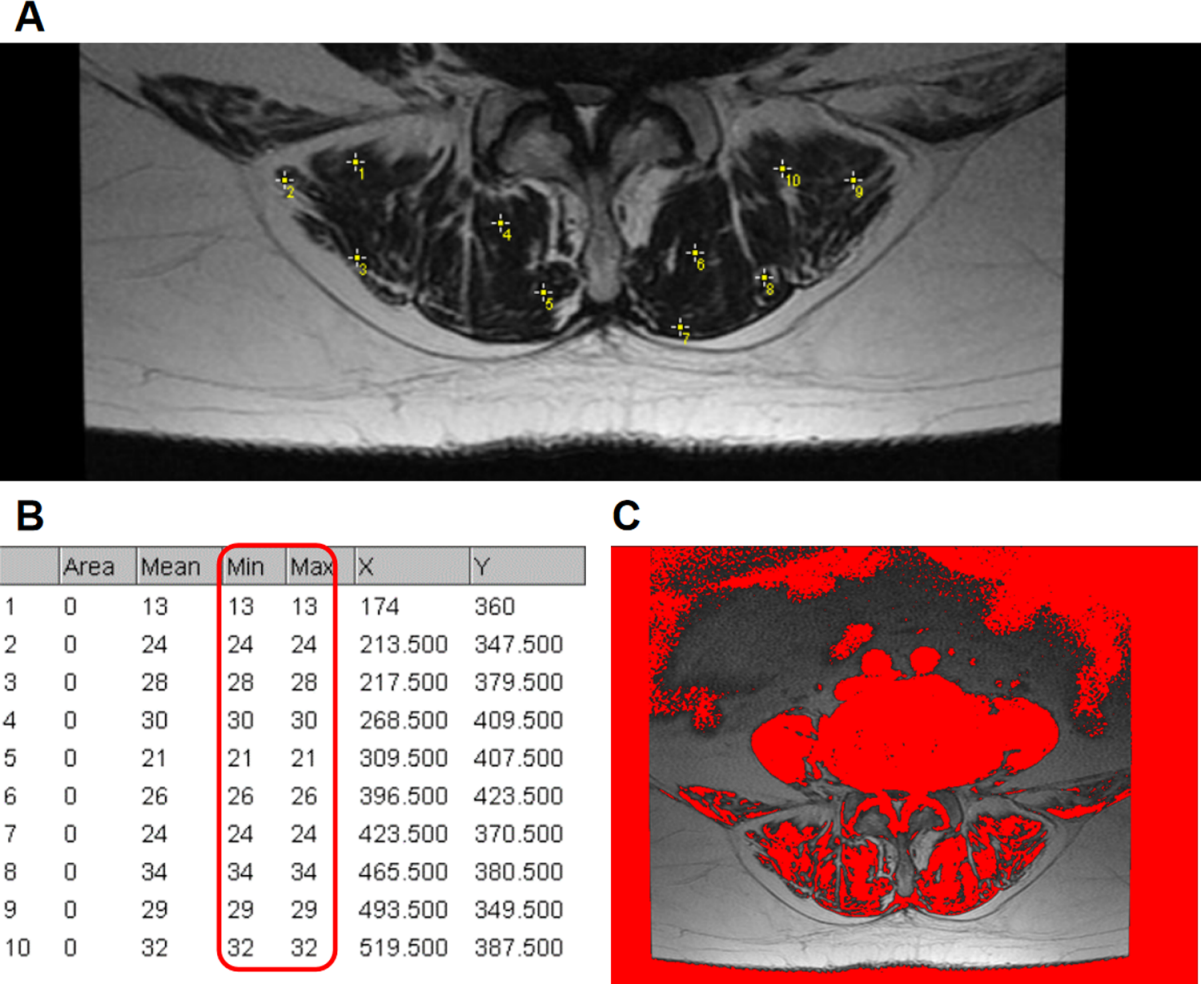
Use of the “Multi-point” tool to determine the threshold to distinguish lean muscle tissue from fat tissue. (A) Ten sample points without any visible pixel of fat tissue within the bilateral paraspinal muscle were selected. (B) The maximum signal intensity of the ten points was determined as the threshold. (C) An example axial T2 weighted MR image of the functional cross-sectional area of the muscles (red area), using the threshold method.
